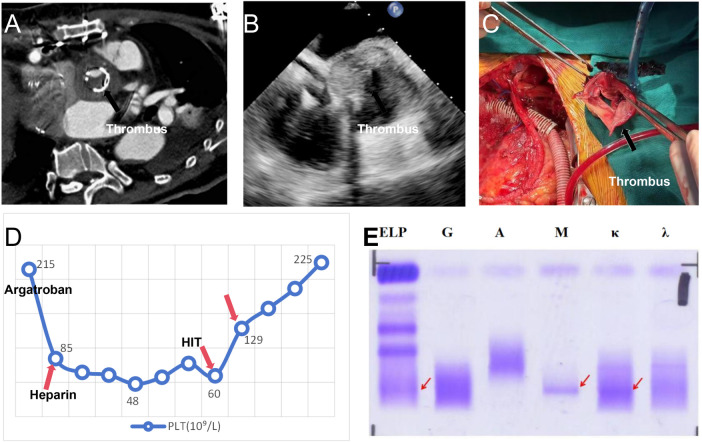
Imaging and laboratory findings of HIT with aortic valve thrombosis. (A) CTA demonstrates thrombus formation on the bioprosthetic aortic valve (black arrowhead). (B) The TEE shows thrombus formation on the bioprosthetic aortic valve (black arrowhead). (C) Intraoperatively retrieved white thrombus (black arrowhead). (D) Time-dependent changes in platelet count following heparin exposure and argatroban initiation. (E) Serum Immunofixation Electrophoresis. The electrophoretogram demonstrates an IgM-κ type monoclonal immunoglobulin. Monoclonal immunoglobulin band (indicated by the red arrowhead).
